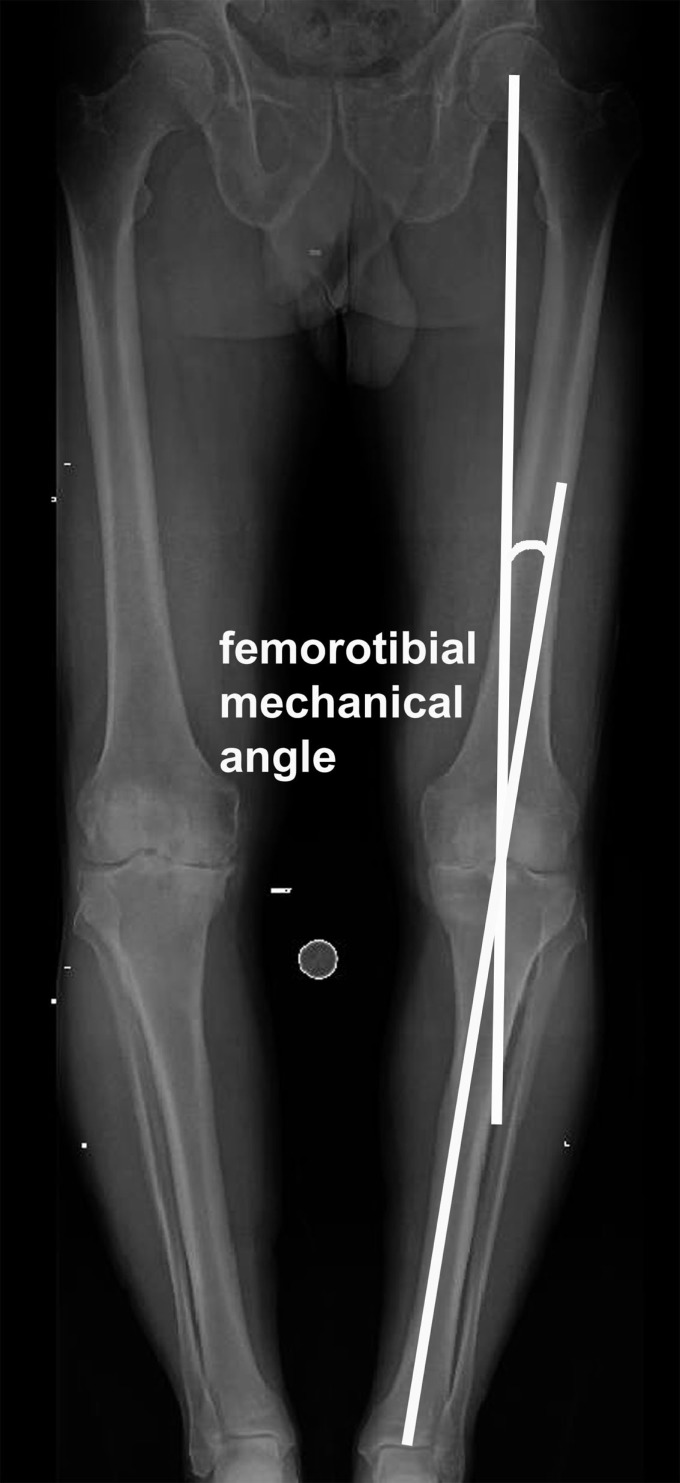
The method of alignment measurement on plain radiographs.
